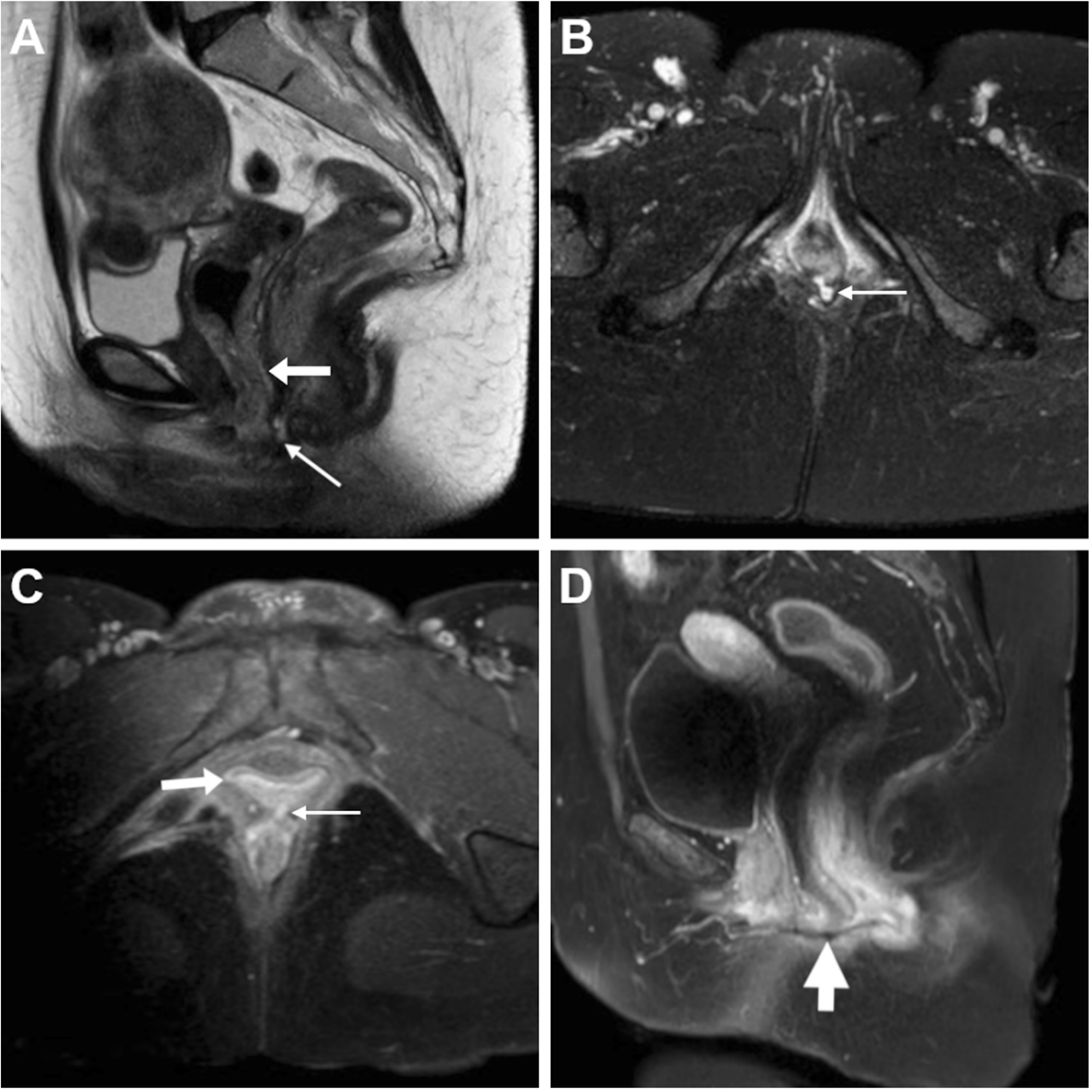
MRI of uncomplicated perianal Crohn’s disease (CD). a, b in a 41-year-old, the ano-VF is seen as a midline fluid-filled track (thin arrows) coursing through the anovaginal septum to reach the lower third of the vagina (arrow) on sagittal (a) and FS axial (b) T2-weighted images. c, d in a 36-year-old with colonic CD, the ano-VF is initially seen as a thin midline tract (thin arrow) on axial FS T1-weighted image after gadolinium CM (c) that reaches the vagina (arrow). On infliximab, repeated MRI including sagittal FS T1-weighted image after gadolinium CM (d) shows positioning of a seton (thick arrow) through the ano-VF
